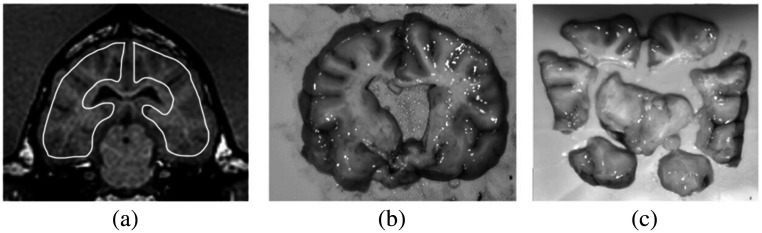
(a) Representative images for hemispheric ROIs on one of three slices drawn on IVIM and on DSC on an anatomic T1 weighted MRI. Edges are avoided to avoid subarachnoid CSF and DSC susceptibility artifacts. The brain slice (b) is cut into eight regions (c) for microsphere analysis and averaged for hemispheric qCBF.
